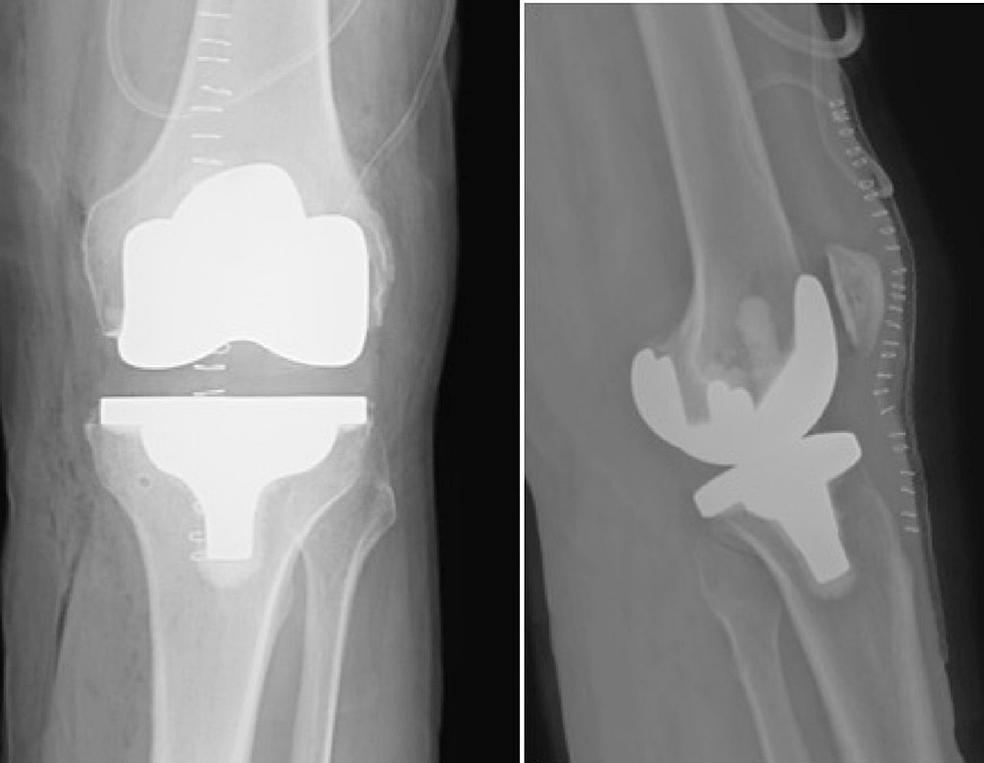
Anteroposterior (left) and lateral (right) radiographs of the immediate postoperative primary total knee arthroplasty.
